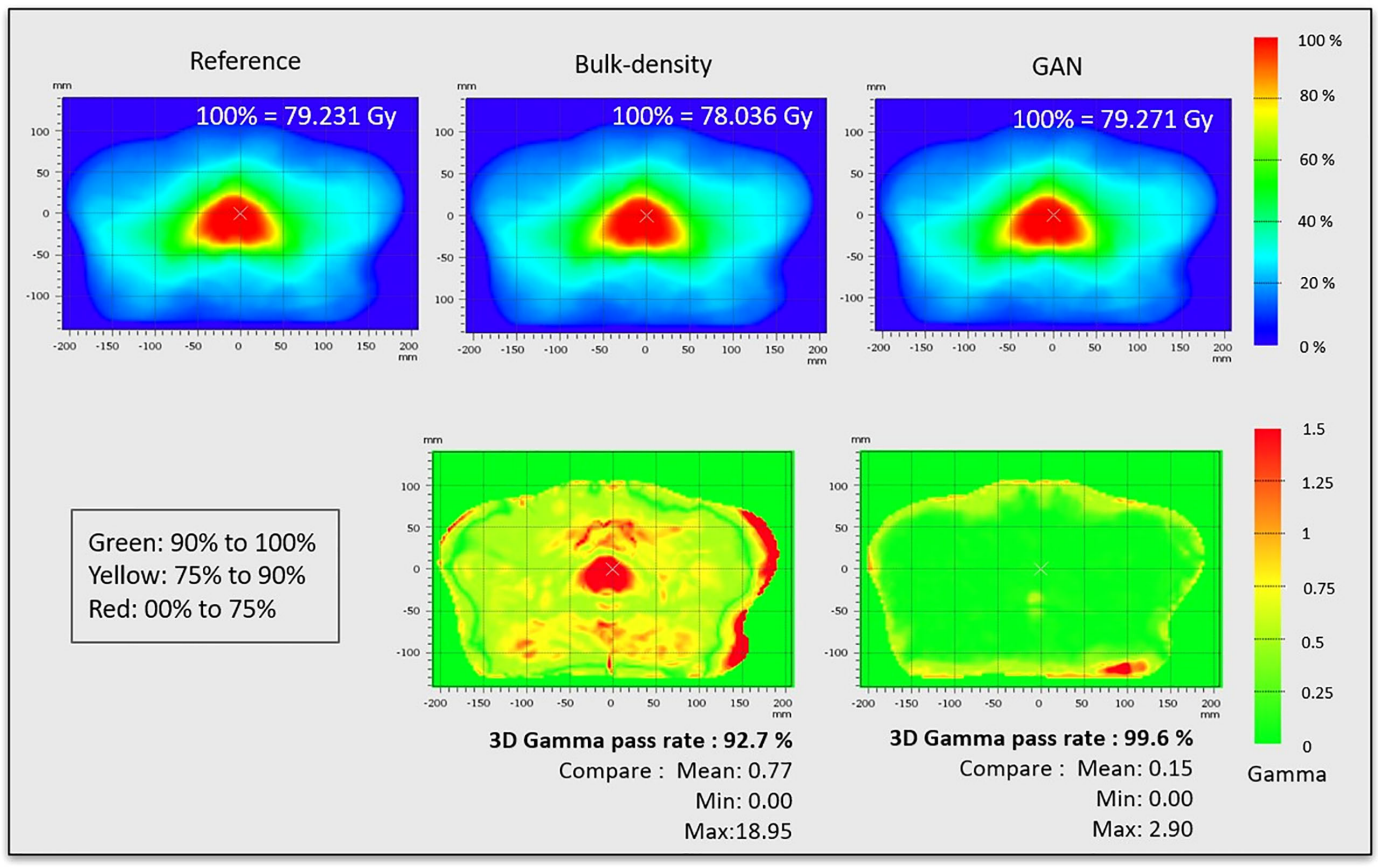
Dose distributions and gamma maps. Dose distributions were propagated to the CCS and combined, resulting in mean reference CT dose, mean dose for sCT generated from bulk density, and mean dose for sCT generated from GAN method. These dose distributions were used to calculate the gamma pass rate (criteria: 3D, local, 1%/1 mm, low dose threshold = 10%).
